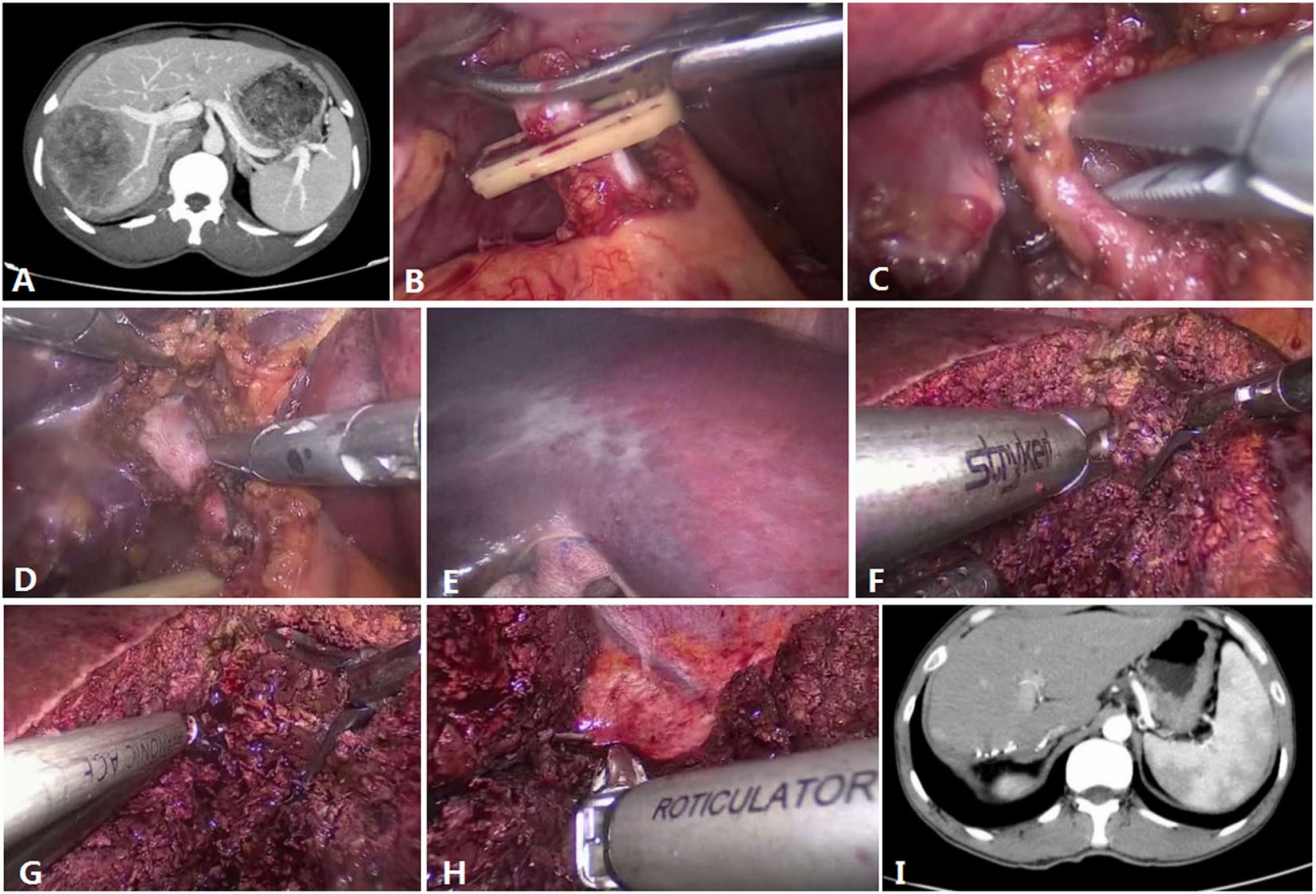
Surgical techniques for LRH. (A) the preoperative CT film of patient; (B) The cystic duct was dissected and ligated; (C) The right hepatic artery were dissected and then ligated. (D) The right portal vein was dissected and was ligated. (E) The hepatic ischemic line; the left intrahepatic bile ducts are interrupted and the visible stones are removed. (F,G) Intrahepatic duct isolated and ligated; (H) The right hepatic vein and the surrounding parenchymal tissues are transected using an Endo-GIA device. (I) The CT film of patient after 1 month.
